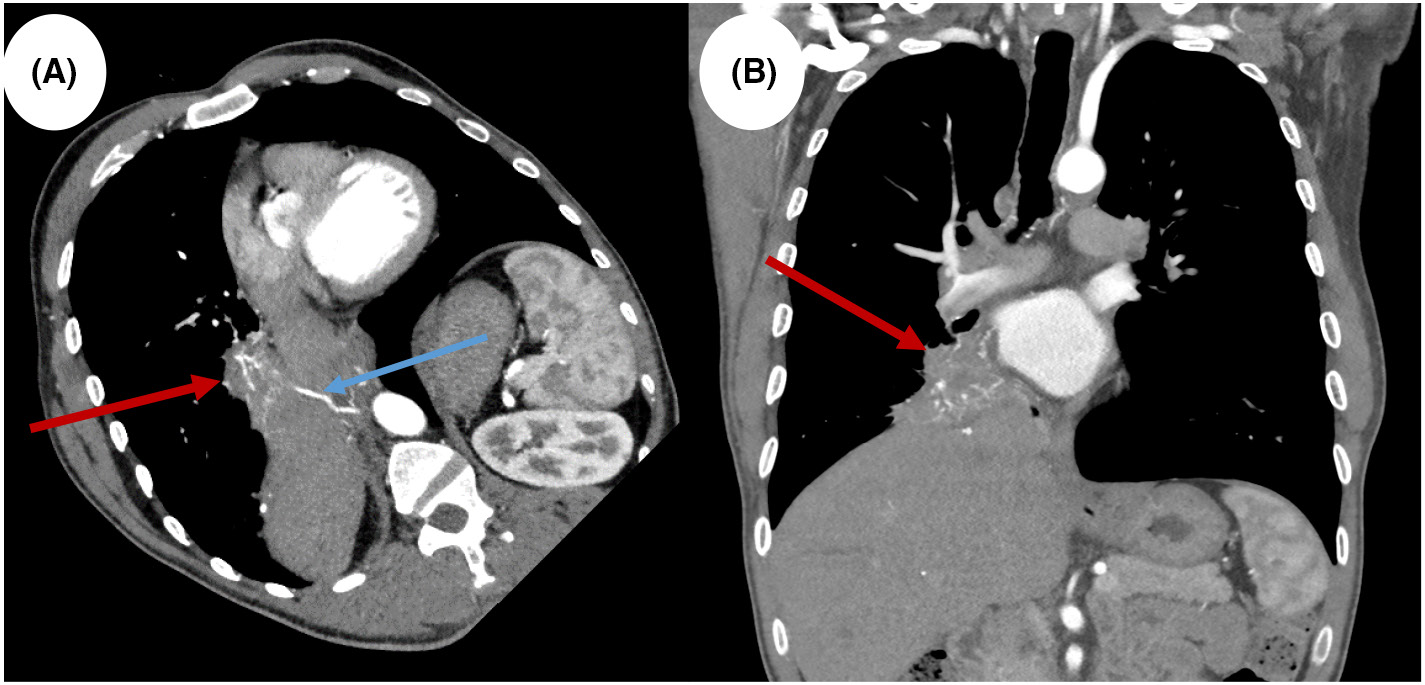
Oblique reconstruction (A) and coronal (B) computed tomography views demonstrate a large irregular heterogenous lesion in the inferior and medial aspect of the right lower lobe (red arrows), measures about 7.4 × 4.6 × 6 cm as anteroposterior (AP), transverse (TR), and craniocaudal (CC) diameters respectively with an anomalous arterial supply (blue arrow).
